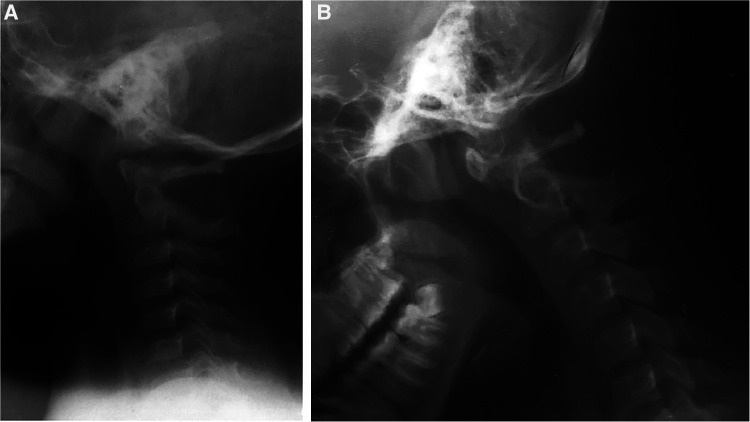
(A) Lateral cervical spine radiograph after motor vehicle accident of a 4-year-old girl, in 1986. Note the widening of C1–C2 interspinous space and the intact odontoid process. (B) Lateral cervical spine X-ray obtained 10 years later [patient in (A)]. There is a C1–C2 dislocation and os odontoideum.
